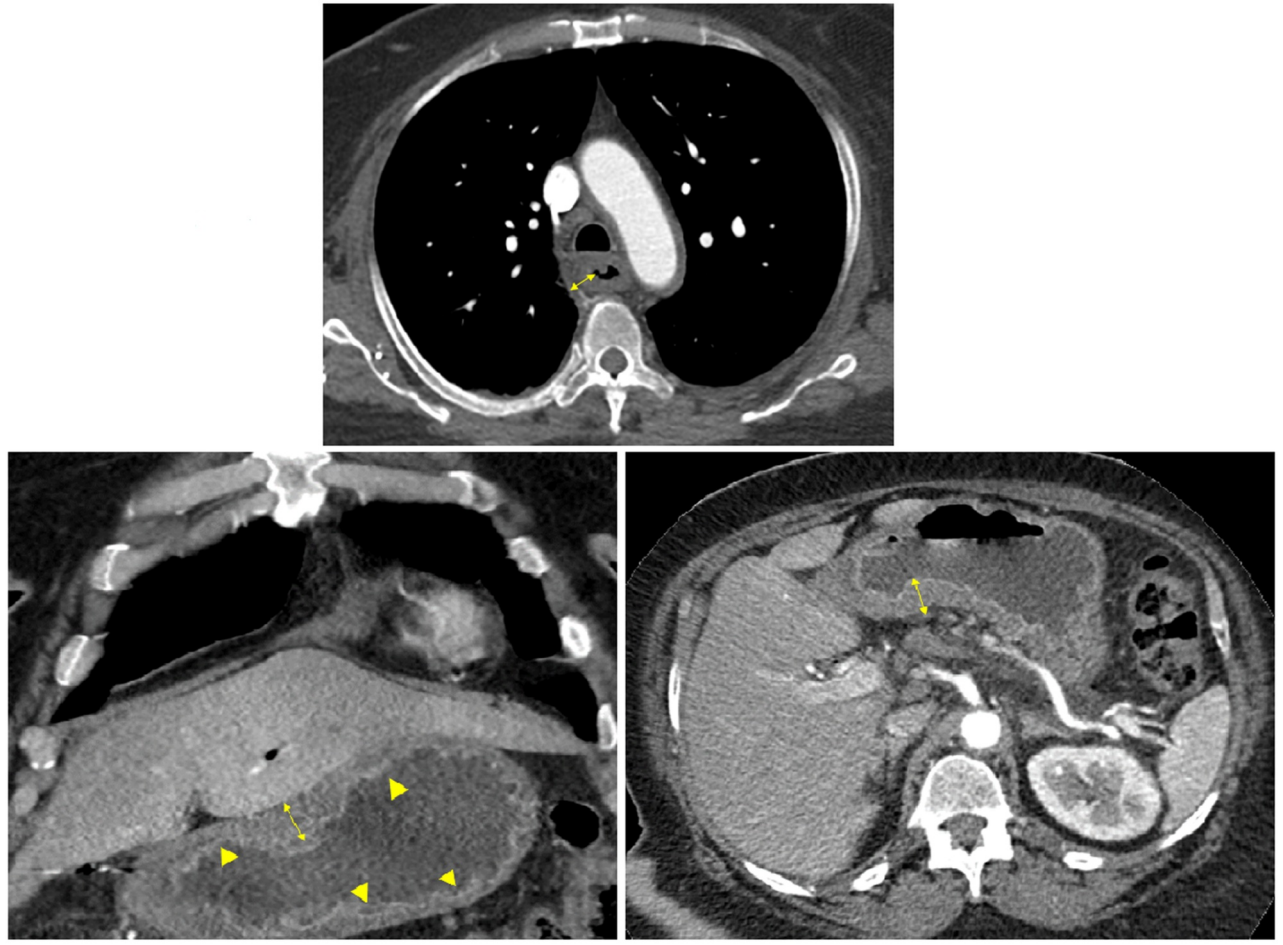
CT of chest and abdomen Upper image: long segment circumferential thickening of the esophagus representing esophagitis (yellow double arrows). Lower images: diffuse gastric wall thickening and mucosal enhancement representing gastritis (yellow arrowheads, and double arrows).
